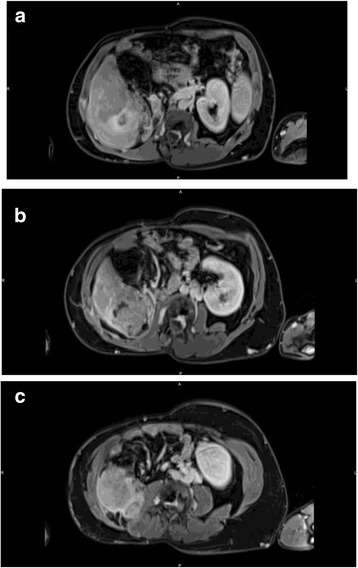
Venous phase CT scanner showing a right flank tumor invading segment VI of the liver (a), right kidney fossa, anterior sheet of right psoas muscle and right colon (b and c)
